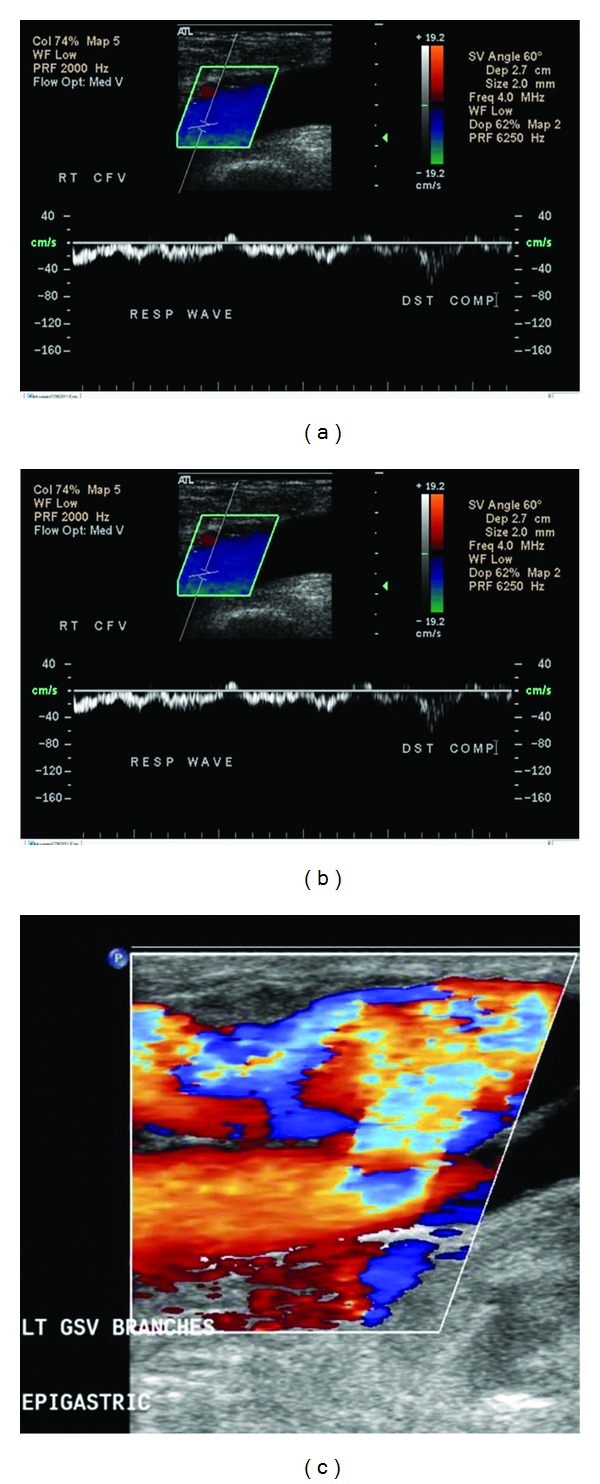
(a) Normal-to-high signal in the left common femoral vein 3 years before presentation. (b) Increased Doppler velocity in the left common femoral vein 1 year prior to presentation. (c) Marked turbulence and high flow at the junction of the left great saphenous vein with the femoral vein.
